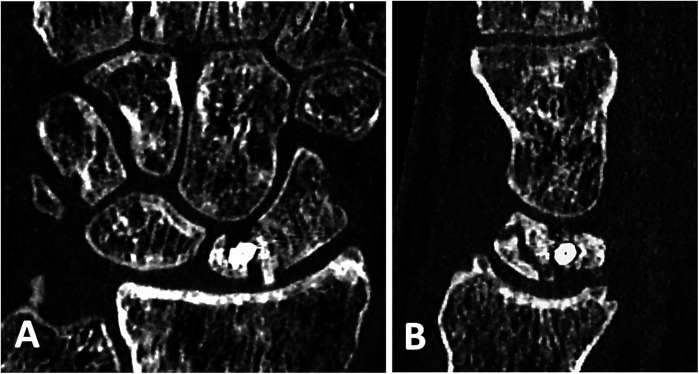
Preoperative CT scans of the patients 5 months after a failed scaphoid reconstruction with a cortico-cancellous bone graft from the iliac crest and a compression screw showing a site of nonunion: (A) in coronal and (B) in sagittal projections. Scaphoid reconstruction has been performed (See Figure 4).
